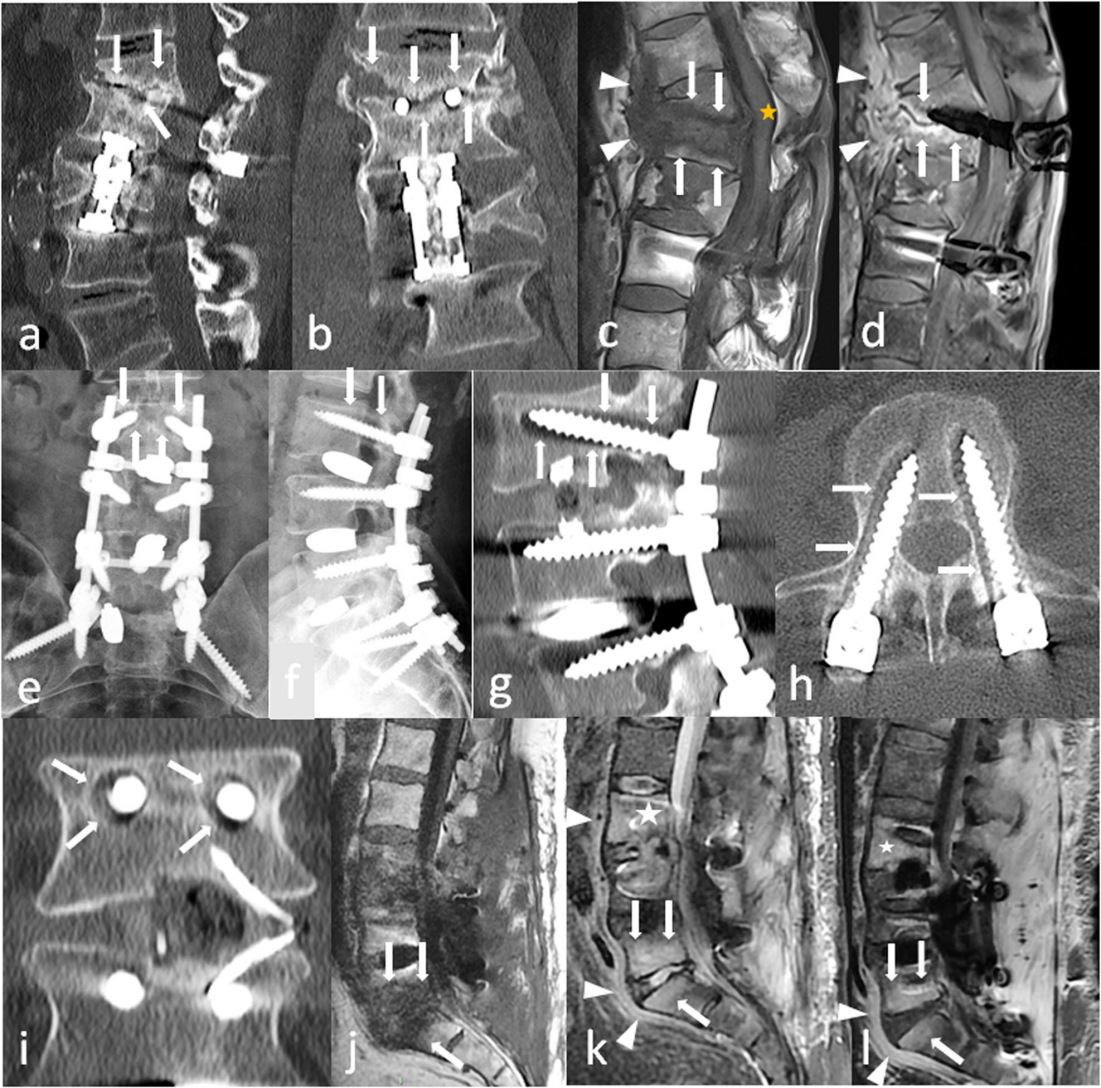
a–d Patient follow-up few months after a T12–L2 fusion procedure for a L1 split fracture with persistent back pain and inflammatory syndrome. Sagittal (a) and coronal (b) CT reconstructions show a T11–T12 large vertebral body resorption with irregular margins and associated sclerosis (arrows) with kyphotic deformation. On coronal images, T12 screws are within the disk. On complimentary MRI with sagittal T1- (c) and post-contrast fat-suppressed T1 images (d), oedema (arrows) of T11 and T12 bodies and frankly enhancing prevertebral soft tissue thickening (arrowheads) confirm the diagnostic of postoperative spondylodiscitis with T11 body resorption. e–i A different patient complained of persistent pain 6 months after a posterior lumbo-sacral arthrodesis. On anteroposterior (e) and lateral (f) X-rays, and on sagittal (g), axial (d) and coronal (h) CT reconstruction, an extensive osteolysis is visible around the L3 screws with no sclerotic rim (arrows) and typical « owl eyes » on coronal reconstruction (i) that should suggest septic pseudarthrosis. Note the absence of equivalent abnormality around the L4 screws. j–l This other patient suffered from post-operative back pain with inflammatory syndrome after an L3–S1 fusion procedure. MR images reveal extended peridiscal oedema in L5–S1 (arrows), with prevertebral inflammation (arrowheads), which correspond to a spondylodiscitis. Oedema in L3 vertebral body (asterisk), raised suspicion of extended infection in this context, especially since prevertebral tissue show inflammation (arrowhead). Yet one must keep in mind that the meaning of vertebral body oedema alone is debatable
